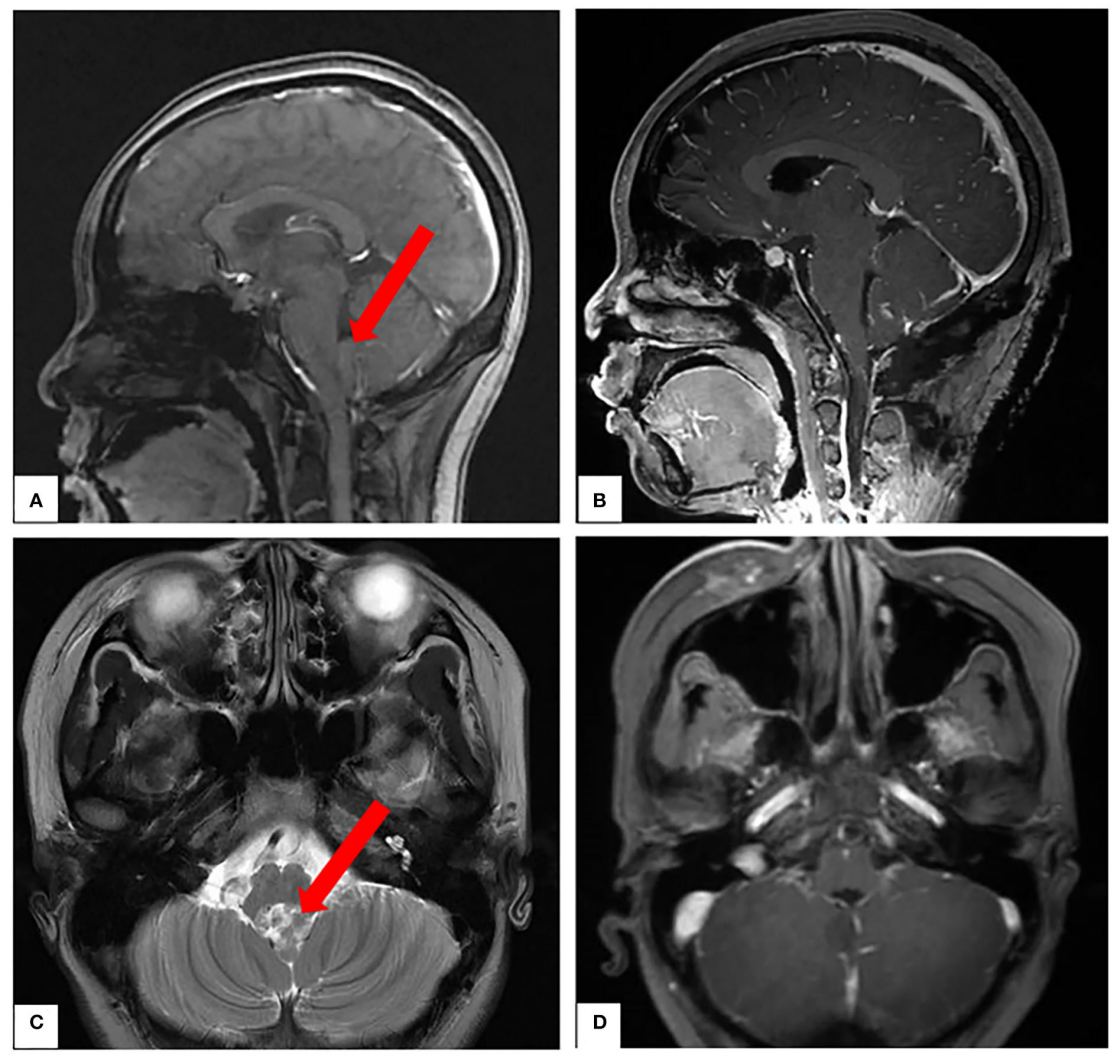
(A,C) Preoperative magnetic resonance imaging (MRI). (B,D) Postoperative MRI.
